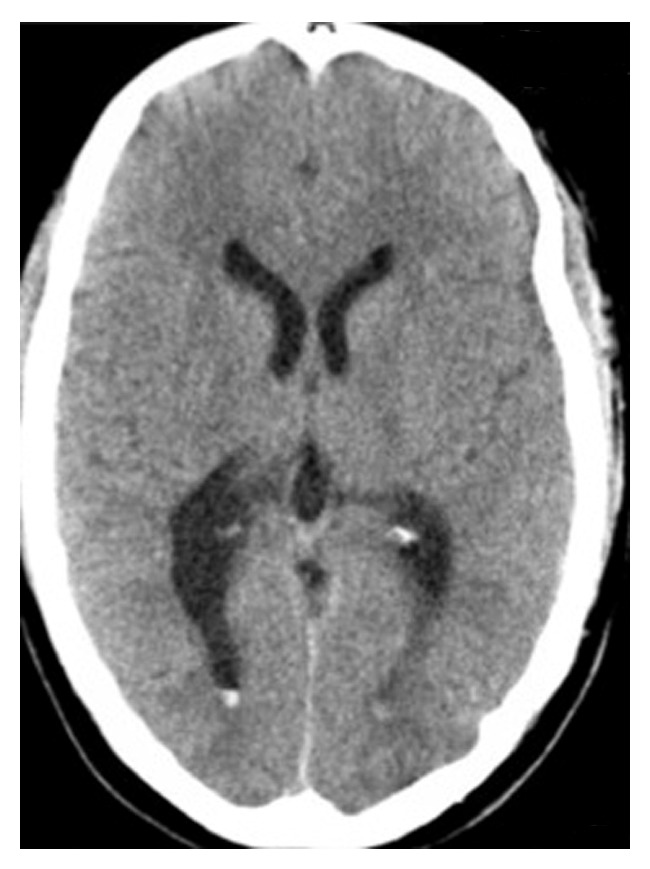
Noncontrast axial computed tomography scan of the head done 3 days after epidural blood patch showing a small amount of blood layering the occipital horns of both lateral ventricles.
